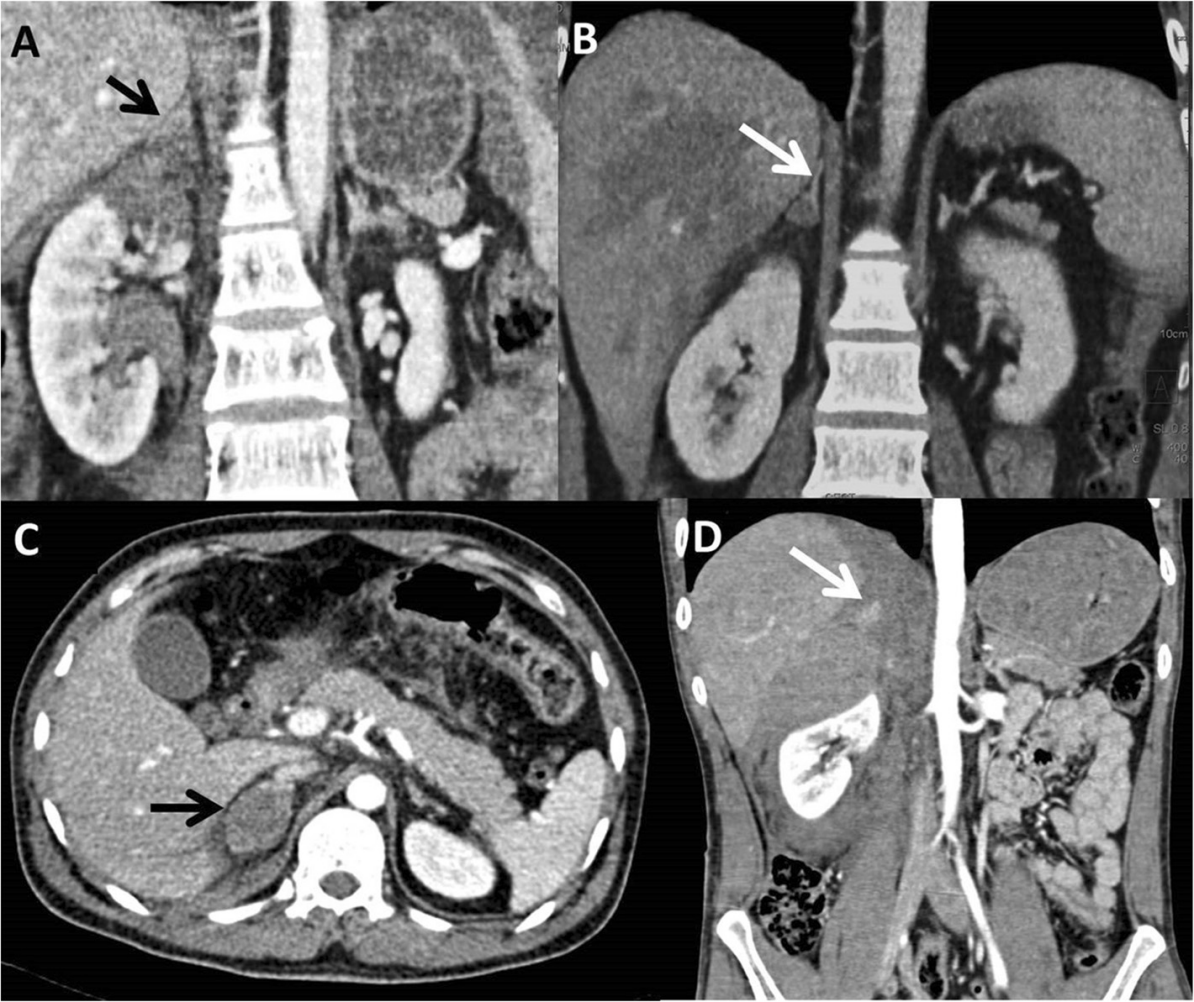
a-d: Various CT appearances of adrenal injuries. CT images show bulky right adrenal gland (a), hematoma splaying limbs (b), oval mass replacing gland (c) and active contrast extravasation in right adrenal gland hematoma (d)
